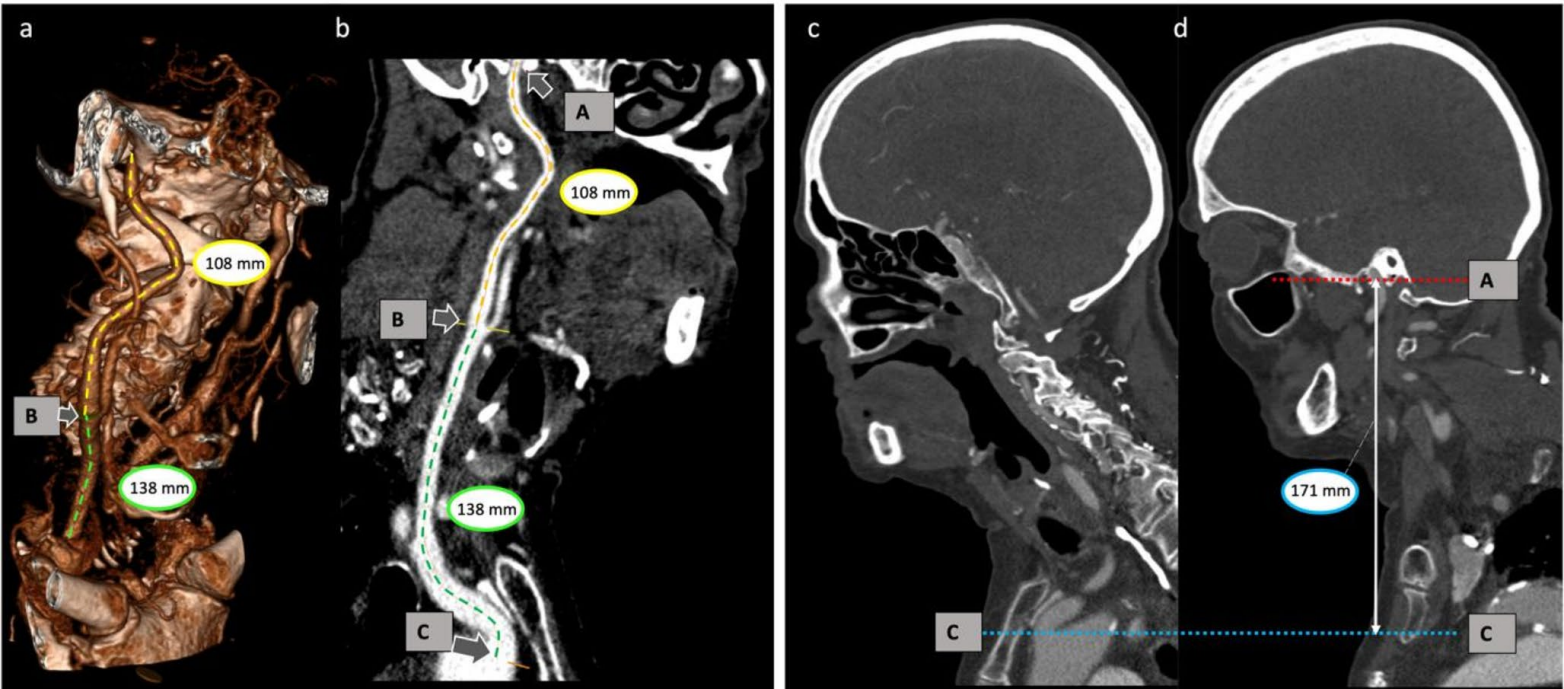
Measurement of Carotid Elongation Ratio. a. & b.: Carotid artery centerline measurement in 3D reconstruction (a) and vascular analysis view (b) using the 3D vessel analysis tool by Sectra AB [Sectra] IDS7/dx version 24.1.13.5511 (x64). Length of common carotid artery (green, 138 mm) and extracranial internal carotid artery (yellow, 108 mm). The total length of the carotid artery (length of the common carotid artery plus the extracranial internal carotid artery) is 108 mm + 138 mm = 246 mm. c. & d.: Z-axis measurement in lateral computed tomography angiography. Blue marker indicates the access artery’s origin from the aortic arch. Red marker indicates the entry point of the internal carotid artery into the skull base. The white line represents the Z-axis between these markers, measured parallel to the CT table. In this case, the Z-axis measures 171 mm. a. - d.: CER from this example: (138 + 108): 171 = 1.43. A: entry point of internal carotid artery into the skull base; B: bifurcation; C: artery’s origin from the aortic arch
